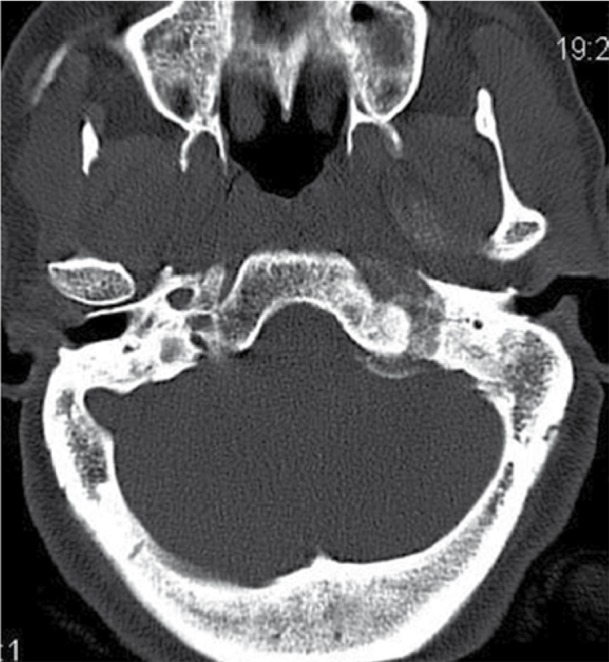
CT Scan Bone Window Shows Irregular Jugular Foramen Well- Defined Soft Tissue Density Mass Lesion in the Left Parapharyngeal Region With Central Dense Amorphous Calcifications. Small Intracranial Part is Also Noted.
